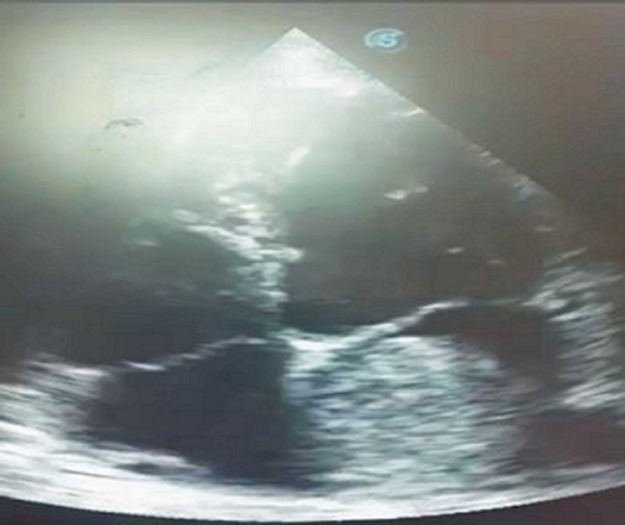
two-dimensional echocardiogram; a large left atrial mass is seen attached to the interatrial septum
